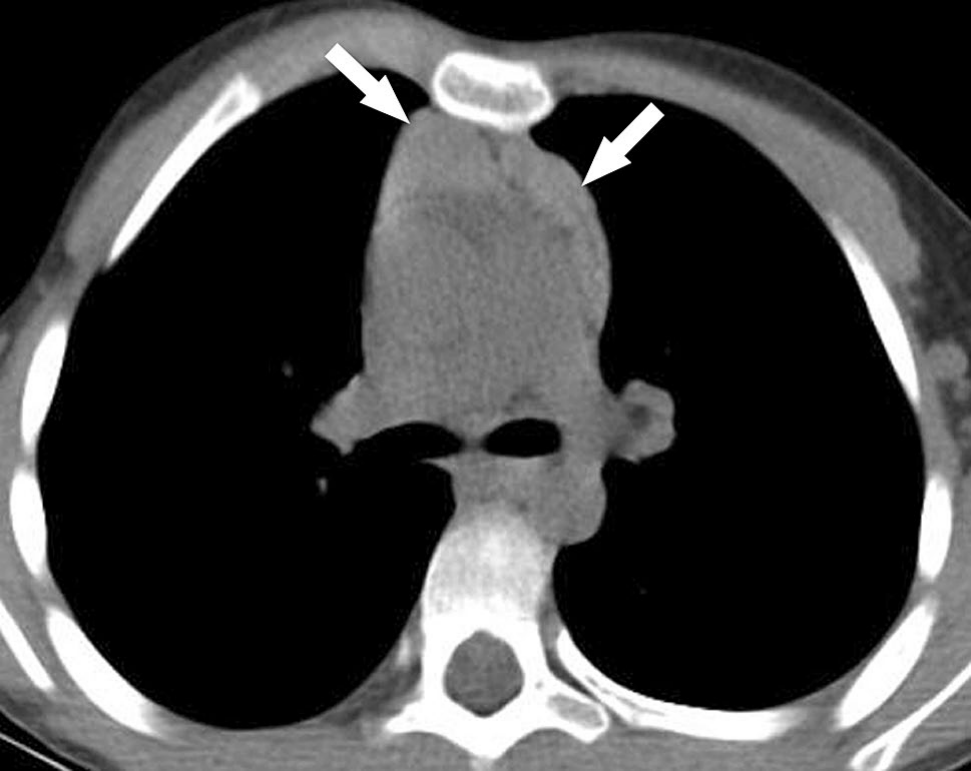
Axial chest CT image in soft tissue windows in a 20-year-old male individual with Proteus syndrome who was otherwise healthy shows an enlarged thymus relative to the individual’s age (arrows).
